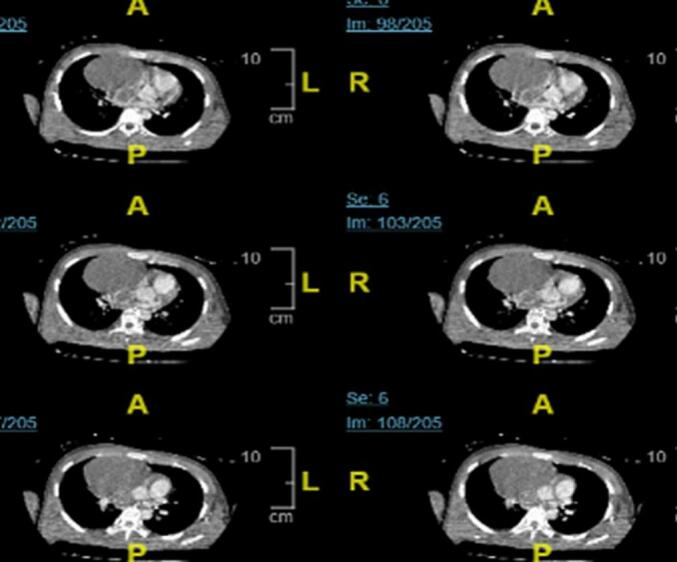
CT scan showed a solid mass in the superoanterior mediastinum, expanding and infiltrating the right atrium.
